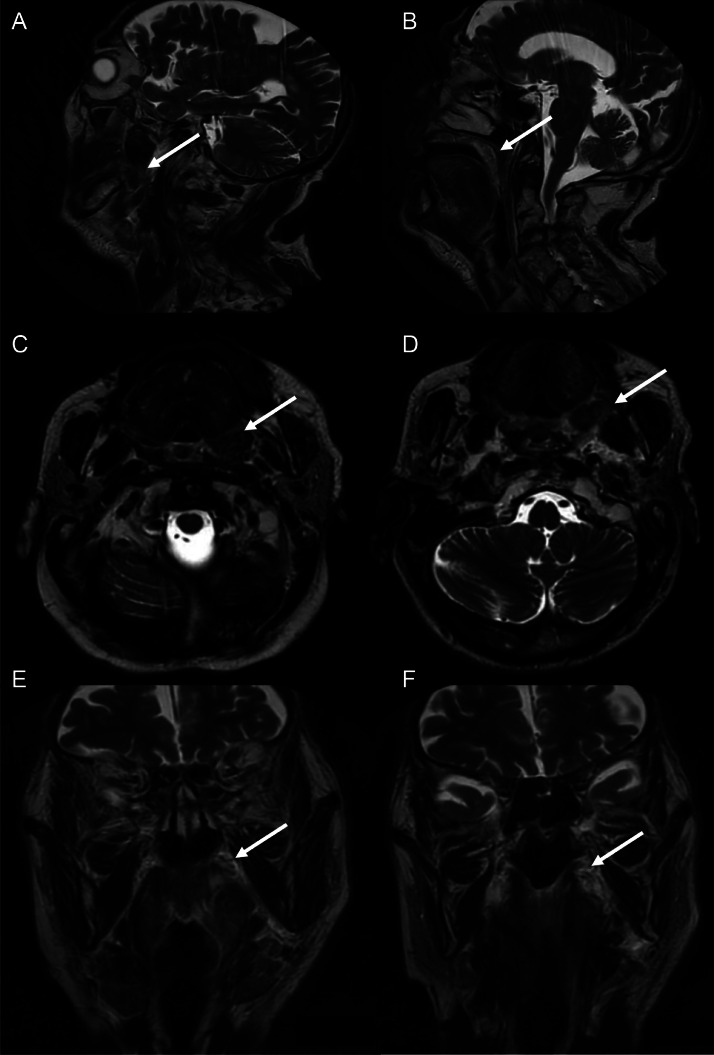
Head and neck MRI of our patient showing partial remission (white arrows). (A and B) Sagittal T2 propeller sequence. (C and D) Axial T2 propeller sequence. (E and F) Coronal T2 propeller sequence.
